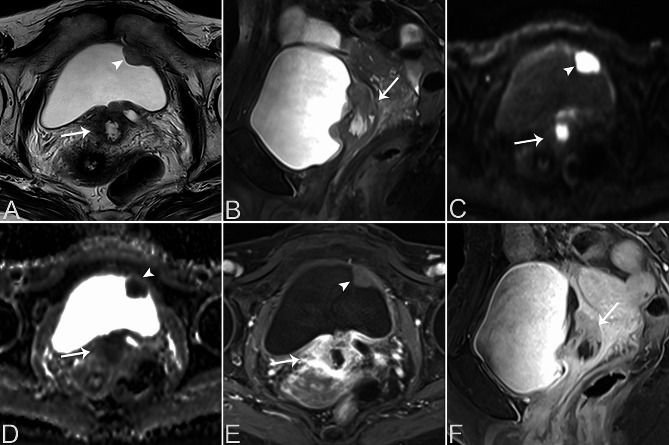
A 61-year-old female patient with bladder SUC in the bladder trigone area and CUC in the left anterior wall of the bladder. Axial T2WI (A) and sagittal T2WI (B) reveal an exophytic mass in the bladder trigone area, demonstrating heterogeneous slight hyperintensity (arrows) with a central area of higher T2 signal indicative of necrosis. The lesion in the trigone area has unclear borders, involving the lower end of the left ureter with ureteral effusion. The lesion of CUC in the left anterior wall of the bladder appears as a nodular mass with slight hyperintensity on T2WI and clear borders (arrowheads). Axial DWI (C) shows both bladder masses with high signal intensity (arrow, arrowhead), and the axial ADC map (D) exhibits marked diffusion restriction (arrow, arrowhead). Contrast-enhanced axial (E) and sagittal (F) T1WI demonstrate heterogeneous and marked enhancement of the trigone area tumor with central necrosis (arrows). The CUC lesion in the left anterior wall of the bladder shows homogeneous mild enhancement (arrowhead)
